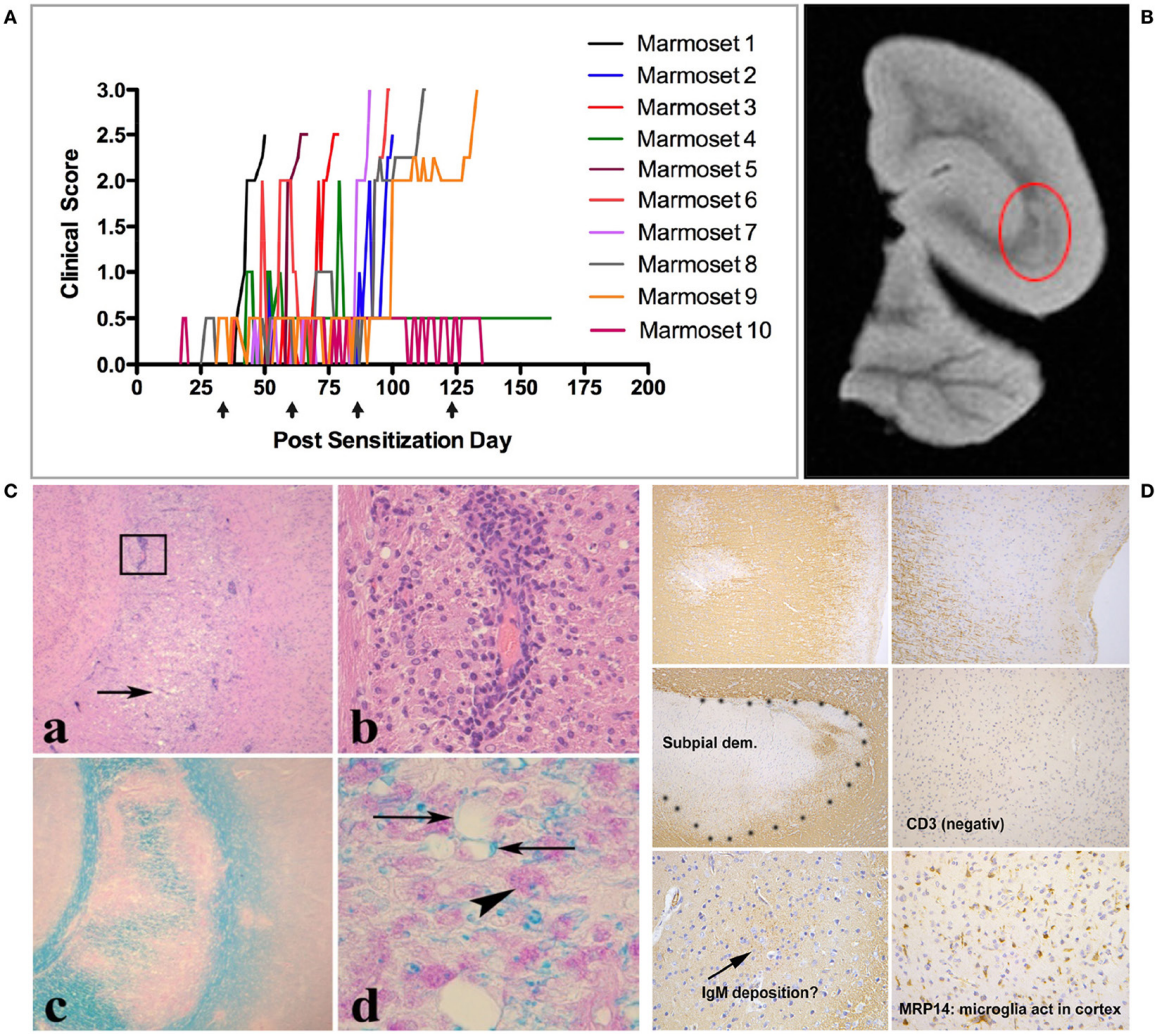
Clinical and pathological aspects of an atypical marmoset experimental autoimmune encephalomyelitis model induced with MOG34–56/incomplete Freund’s adjuvant (IFA). (A) 10 unrelated marmosets were immunized at 28 days interval (arrows) with MOG34–56/IFA. Depicted is the heterogeneous disease development, comprising early and late responders. (B) A representative T2W magnetic resonance images scan of a formalin-fixed brain half, showing multiple hyperintense regions in the white matter. The encircled lesion (red) was examined for presence of inflammation [(C): a,b] and demyelination [(C): c,d]. (D) Demyelinated lesions in the cortical gray matter. In these lesions, mononuclear cell infiltrates and antibody deposition are absent, while prominent activation of microglia is present.
